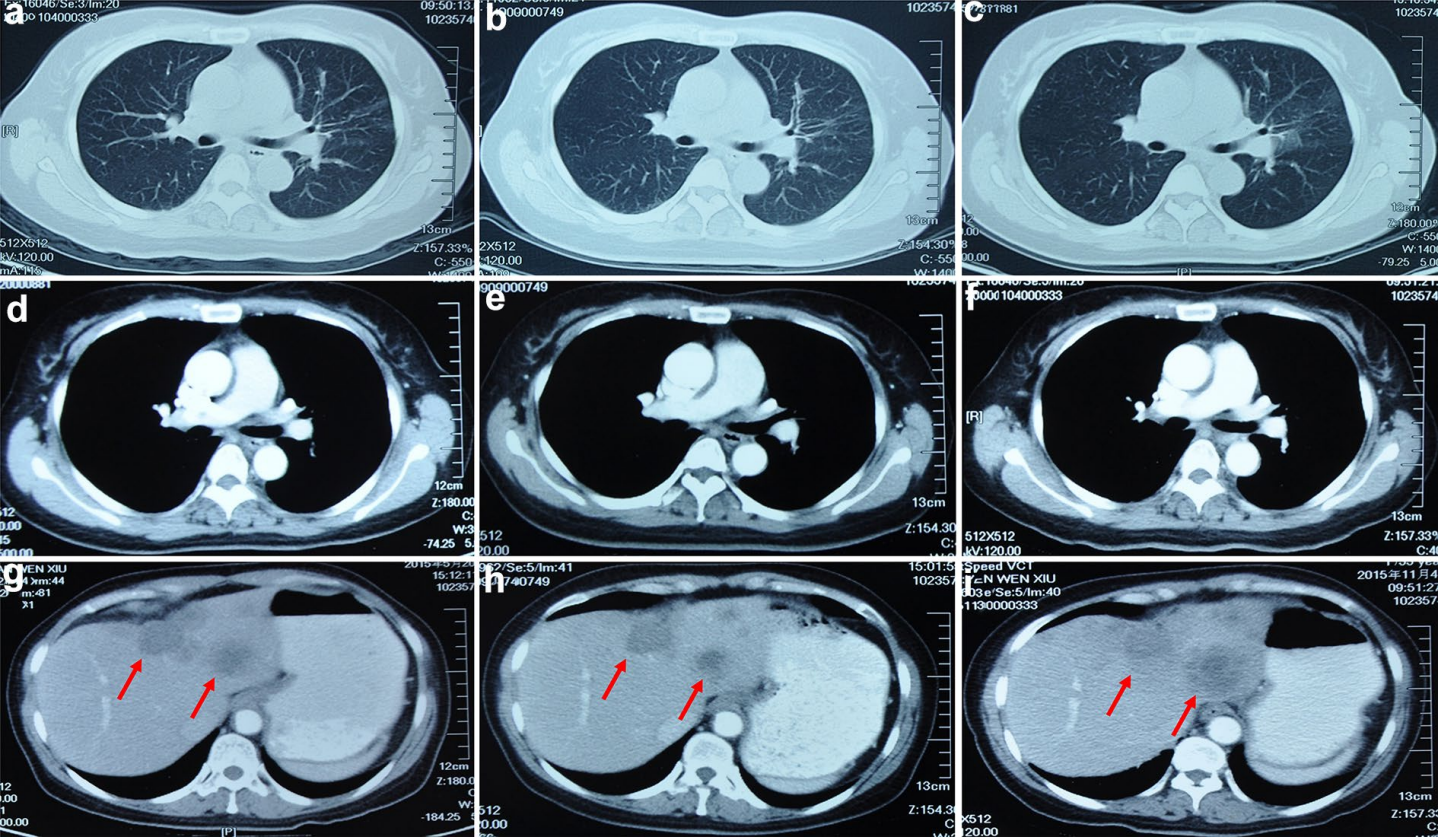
Disease evaluation during the treatment of pemetrexed with bevacizumab. Before treatment of pemetrexed with bevacizumab, liver metastases were assessed using CT scan and no lung lesions were found (a, d, and g). After two cycle therapy of pemetrexed with bevacizumab, metastatic nodule size in liver decreased (partial response) (b, e and h). The CT scan showed the disease progression in liver metastases after eight cycle administration of pemetrexed plus bevacizumab (c, f and i). During the entire course, there were still no metastases in bilateral lungs, mediastinum, brain and so on
